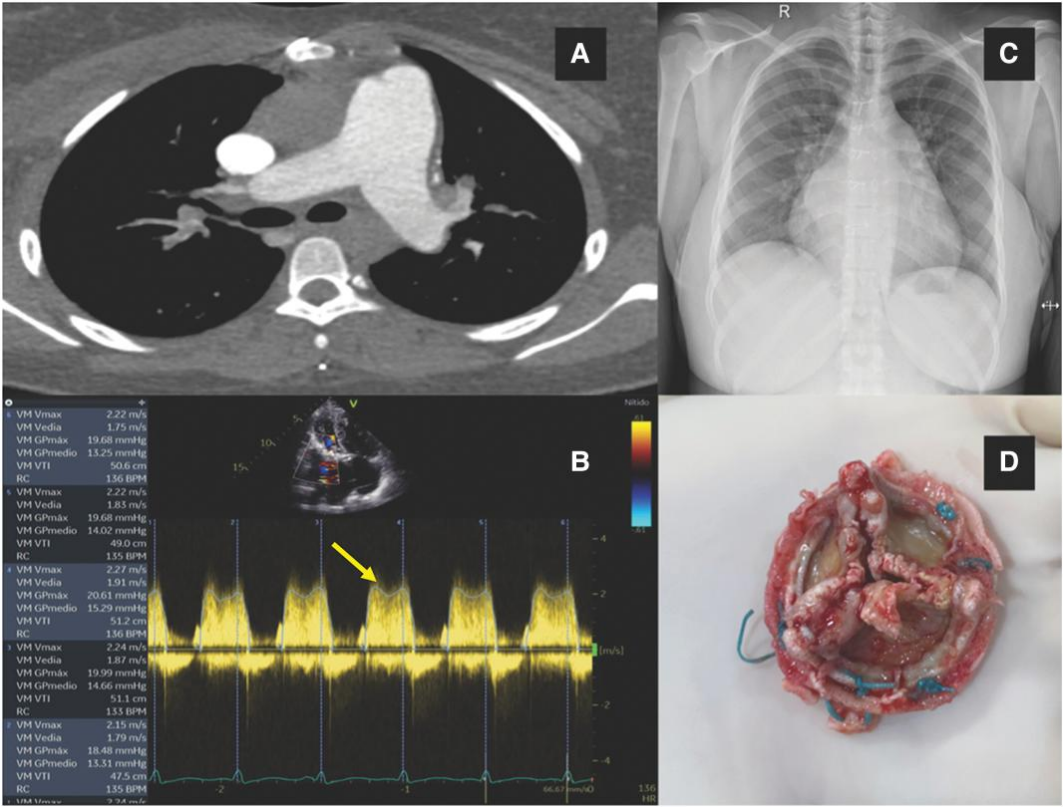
Case 3: (*A*) Pulmonary embolism in the artery for the anterior segment of the left upper lobe and apical region of the right upper lobe. (*B*) Transthoracic echocardiogram in apical four-chamber view. Continuous spectral Doppler of the mitral valve prosthesis (arrow) with high gradients and velocities (maximum velocity of 2.2, mean gradient of 15 mmHg, and mean pressure time of 180 ms). (*C*) Increased cardiac silhouette. Symmetrical pulmonary hila with prominence of vascular markings. (*D*) Explanted mitral valve bioprosthesis showing marked thickening of its leaflets and restriction to opening and closure.
